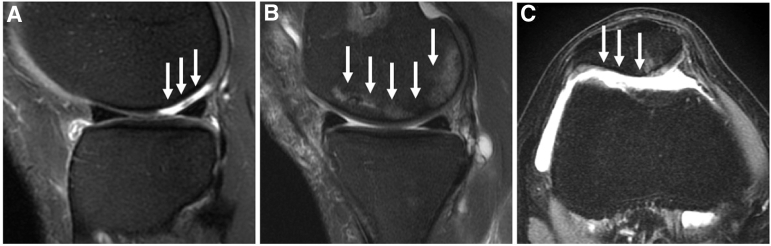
Magnetic resonance imaging findings in patients with high Whole-Organ Magnetic Resonance Imaging Score. (A) Sagittal T2-weighted fast spin echo (FSE) image shows grade 5 cartilage lesion (white arrows) in the lateral femoral condyle. (B) Sagittal T2-weighted FSE image shows grade 3 bone marrow edema-like lesion (white arrows) in the lateral femoral condyle. (C) Axial T2-weighted FSE image shows grade 5 cartilage lesion in the patella (white arrows) with subjacent grade 2 (white arrowheads).
